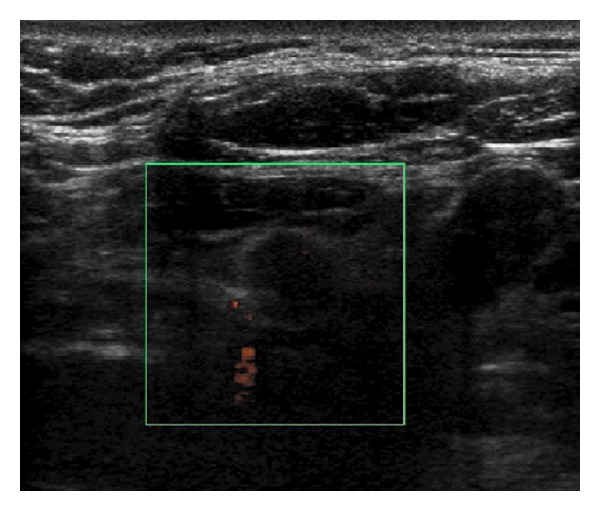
Thyroid ultrasound. Hypoechoic nodule in the inferior pole of the left thyroid lobe.
